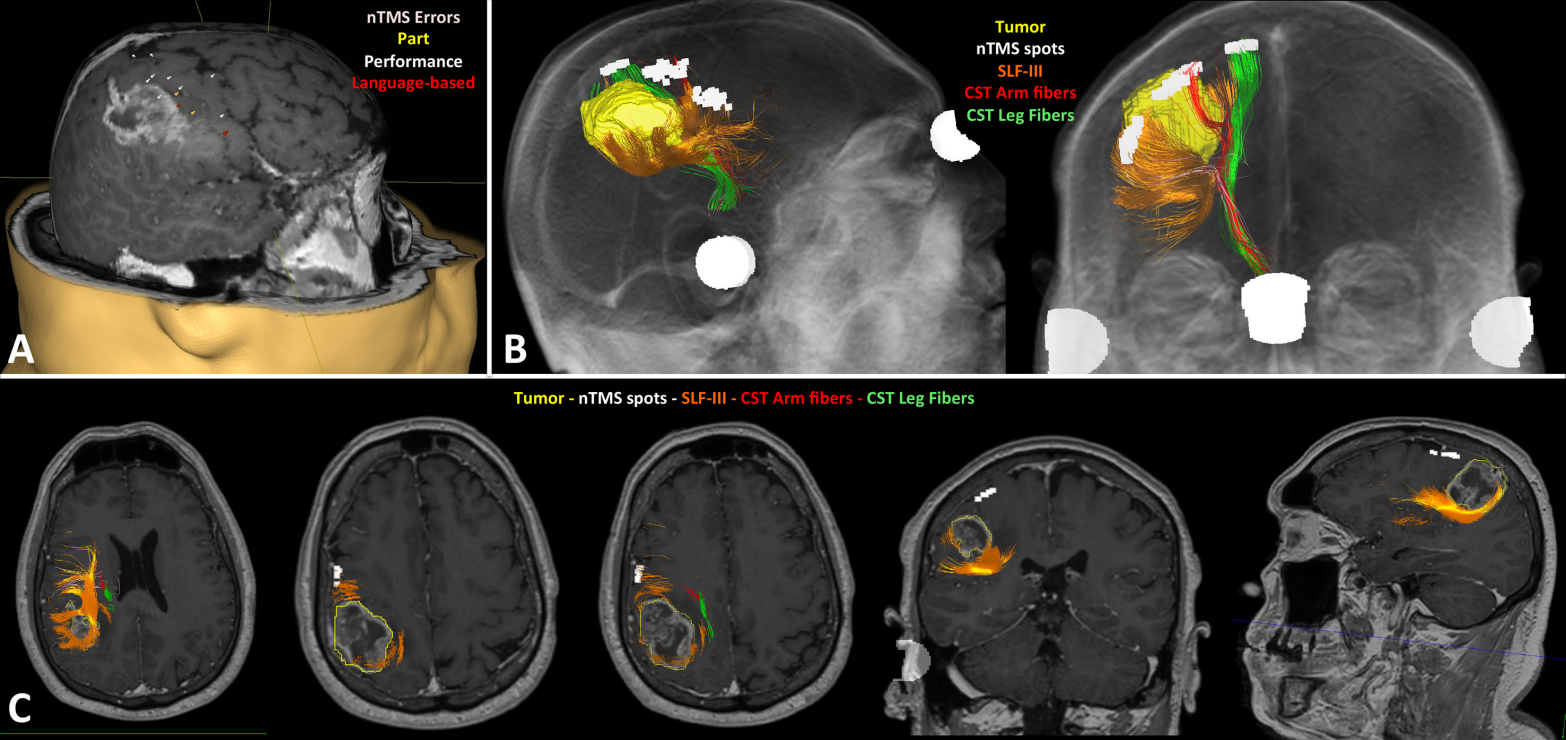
Case example of the preoperative planning in a right fronto-parietal glioblastoma. The nTMS mapping of VSAs identified several eloquent cortical sites of the VS network (A); the DTI tractography showed the tumor (yellow) was very close to the SLF-III (orange), but also to the corticospinal tract (CST; arm fibers in red, leg fibers in green) (B); the fusion MRI scan confirmed the SLF-III was infiltrated by the posterior part of the tumors, as visible in the different axial, coronal and sagittal slices; conversely, the nTMS cortical spots are far away from the tumor (C).
